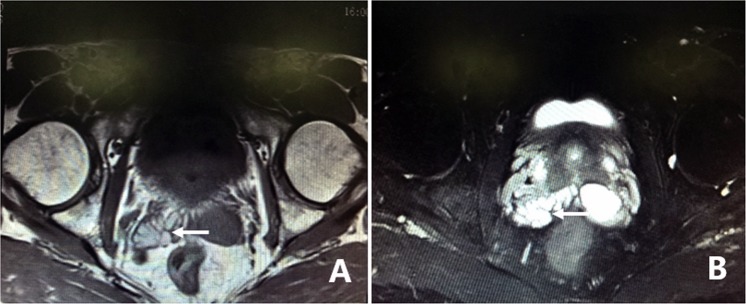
MRI image of an old hemorrhage in the right side of the SV. (A) This image shows high signal intensity in the right SV and low signal intensity in the left SV on T1WI. (B) This image shows similar high signal intensity in both sides of the SV on T2WI. This pattern suggests that an old hemorrhage is present in the right SV. There are slight dilatations and cystic changes in the right and left sides of the SV, respectively.
